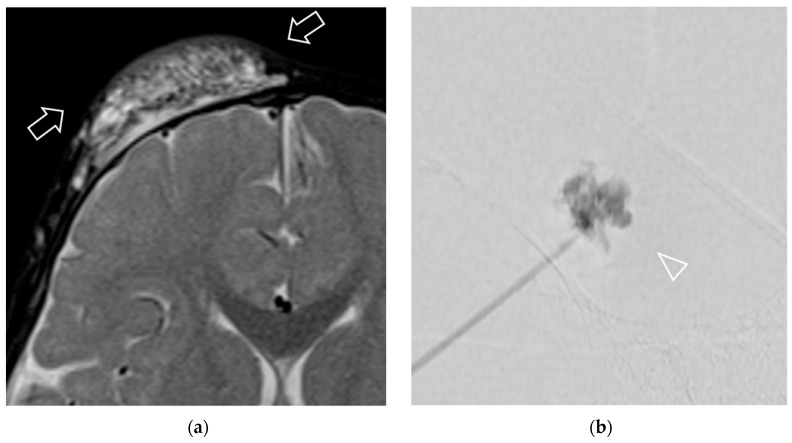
A 12-month-old patient presented with a venous malformation characterized by a palpable mass on the right forehead. The lesion showed non-response to sclerotherapy. (a) A pretreatment axial T2-weighted with fat suppression image revealed a microcystic lesion with high T2 signal (arrows) located at the subcutaneous tissue of right frontal scalp. (b) Pattern of contrast opacification on dynamic digital radiographic images exhibited spongy morphology (arrowhead), and aspirated contents yielded bloody content. She underwent bleomycin injection but showed no improvement in symptoms and no change in size confirmed by physical examination and MRI after 3 sclerotherapy sessions.
